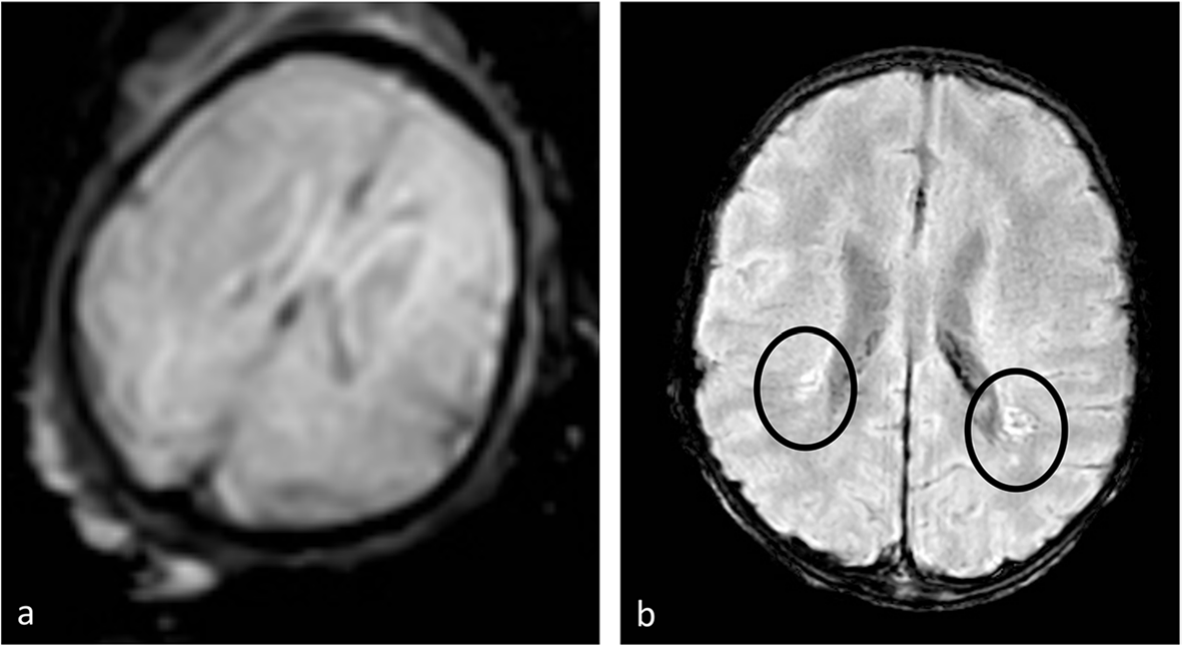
Magnetic resonance imaging. a Prenatal T2-weighted echo planar imaging (EPI) in the axial plane b Postnatal susceptibility weighted imaging (SWI) in the axial plane. Postnatally, the bilateral periventricular calcifications (black circle) are seen, not evident on in utero EPI images
